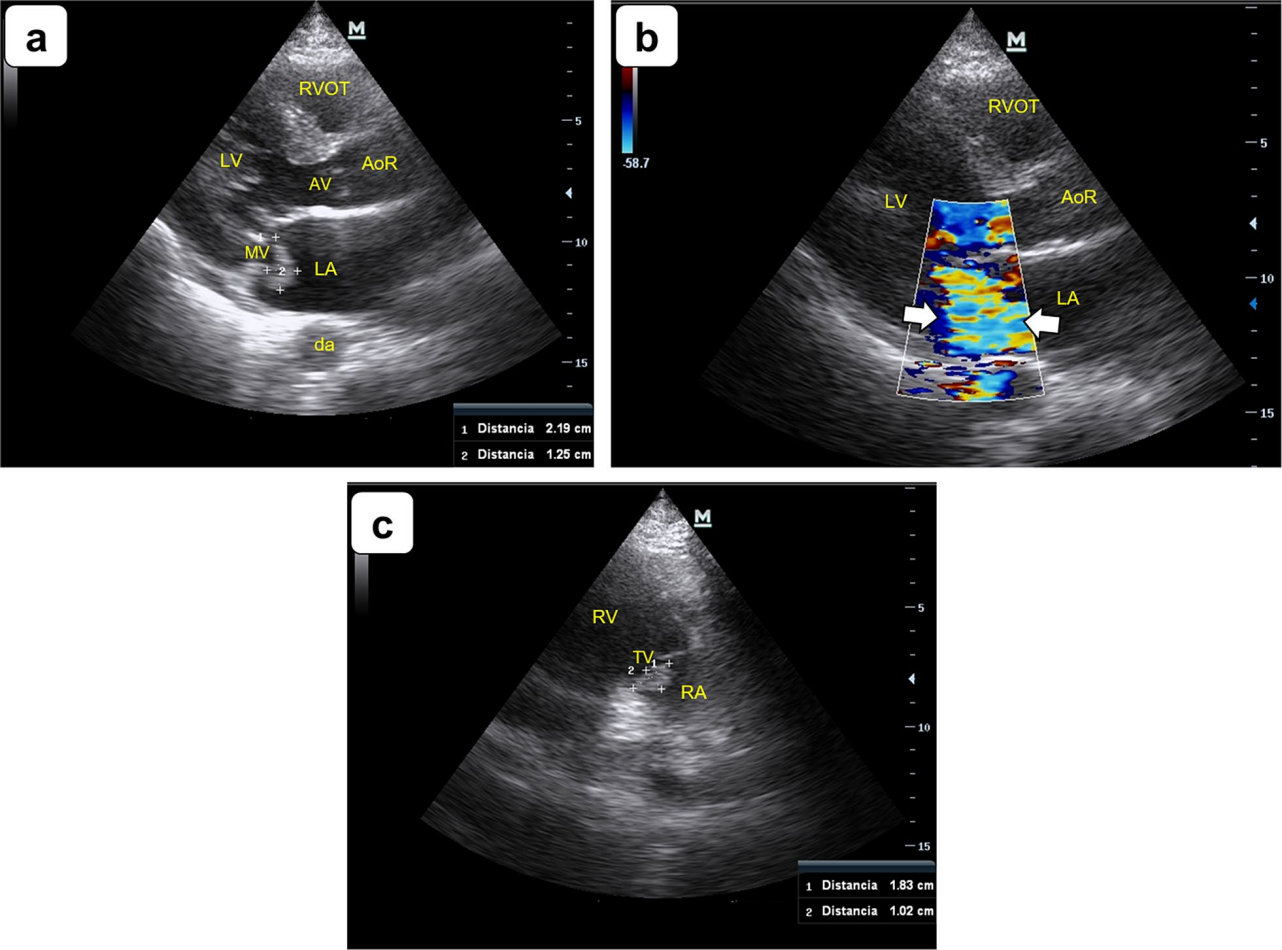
Point-of-care transthoracic echocardiogram showing signs of infective endocarditis. a Parasternal long axis (PLAX) view depicting a vegetation measuring 22 mm × 13 mm (calipers) attached to the atrial side of the anterior leaflet of the mitral valve. b PLAX showing a severe mitral regurgitation (arrows) triggered by the mitral valve vegetation. c Right ventricular inflow view showing a vegetation of 18 mm × 10 mm (calipers) in the atrial side of the posterior leaflet of the tricuspid valve. *LV* left ventricle, *LA* left atrium, *AoR* aortic root, *AV* aortic valve, *MV* mitral valve, *da* descending aorta, *RVOT* right ventricular outflow tract, *RV* right ventricle, *RA* right atrium, *TV* tricuspid valve
